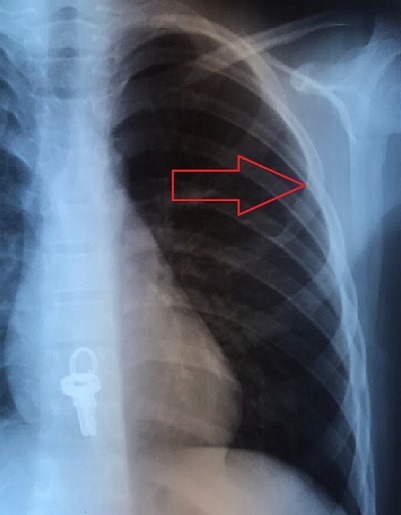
Image de radiographie standard de l'épaule après 4 mois de traitement: on note une reminéralisation de l'omoplate gauche
